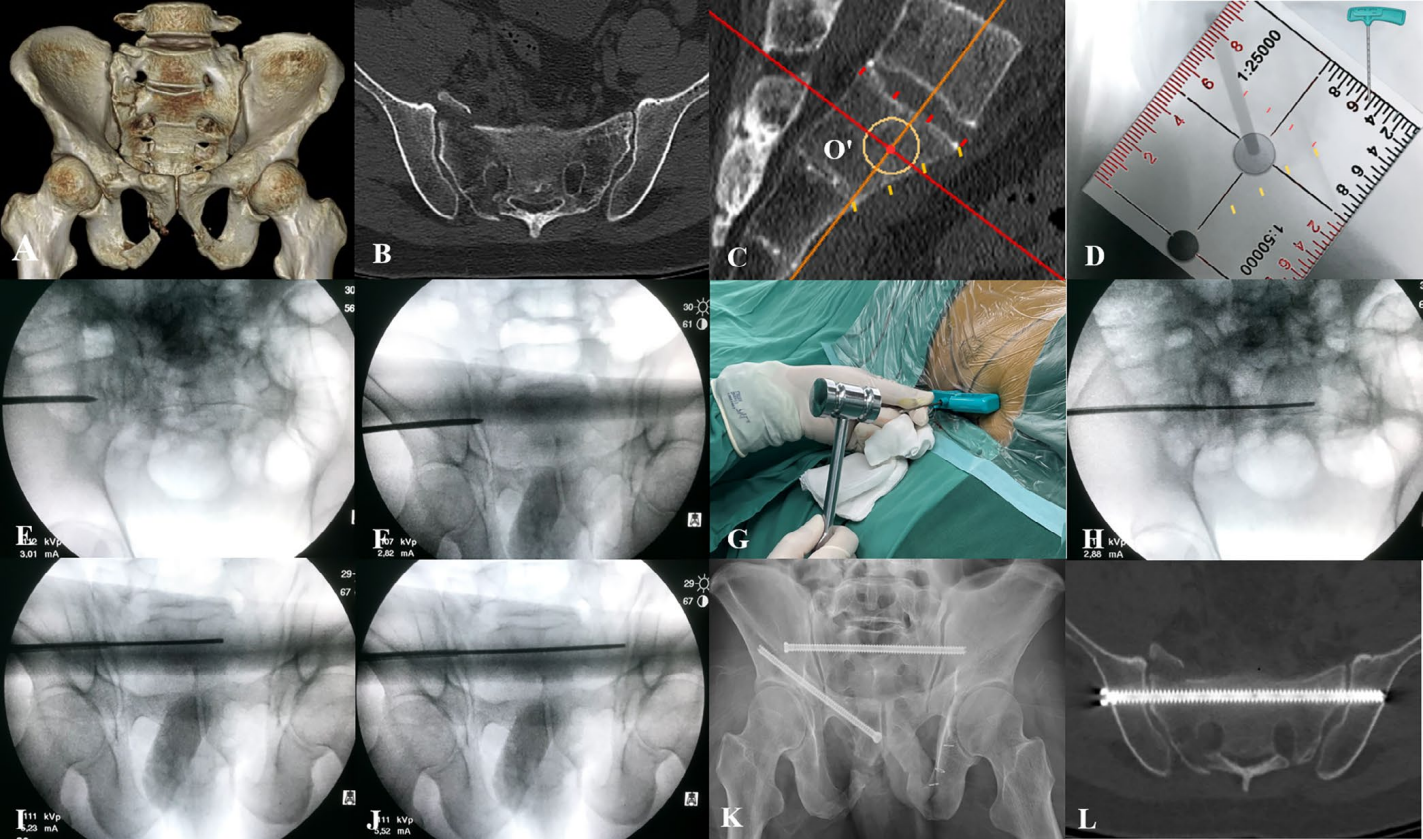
A 41‐year‐old man suffered a minimally displaced pelvic fracture (OTA‐61B2.2) (A, B) The optimal entry point was formulated in the strict mid‐sagittal plane (C), and transferred onto the lateral fluoroscopy (D). A percutaneous kyphoplasty canula was hammered to perforate the outer cortex of the ilium and pass through the ipsilateral sacroiliac joint (E, F). A reversed Kirschner wire was gently hammered and advanced (G–J). Postoperative pelvic radiograph and axial CT image showed the Grade‐1 placement of the transiliac‐transsacral screw (K, L).
